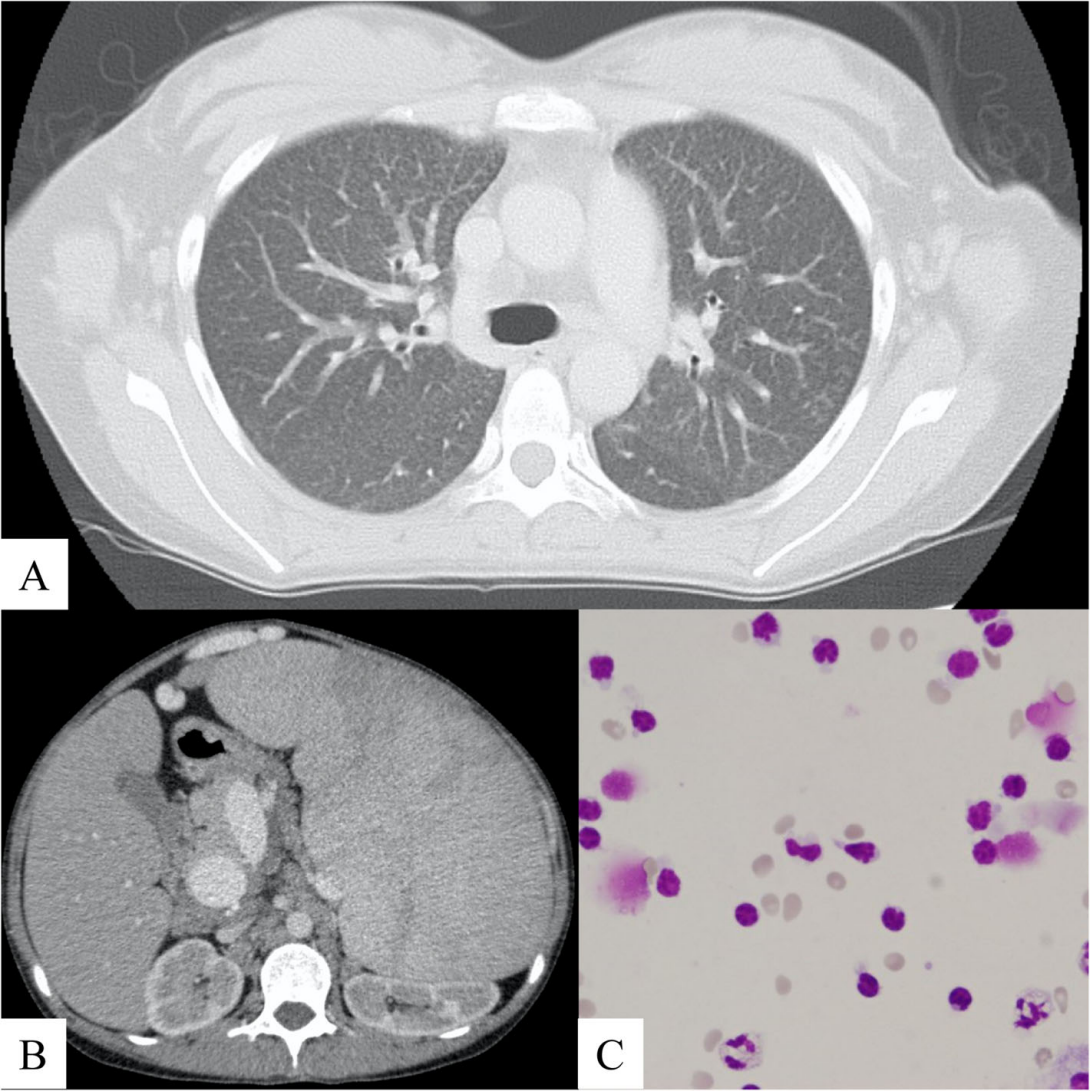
a Chest computed tomography (CT) scan revealed the presence of centrilobular nodules in the lung parenchyma. b Abdominal CT scan revealed the remarkable enlargement of the spleen. c The first analysis of the bronchoalveolar lavage fluid revealed a high proportion of small lymphocytes
